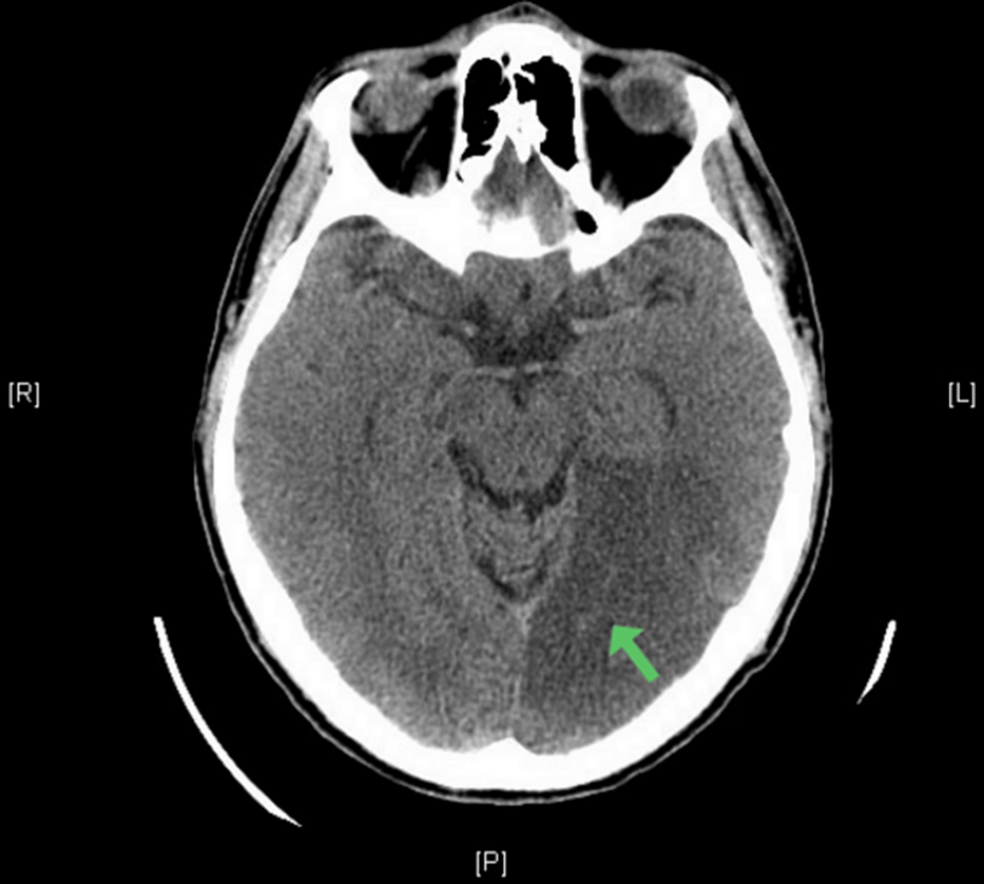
Hypodense occipitotemporal cortical-subcortical lesion on the left side (green arrow) in the contrast-enhanced computed tomography of the brain.
